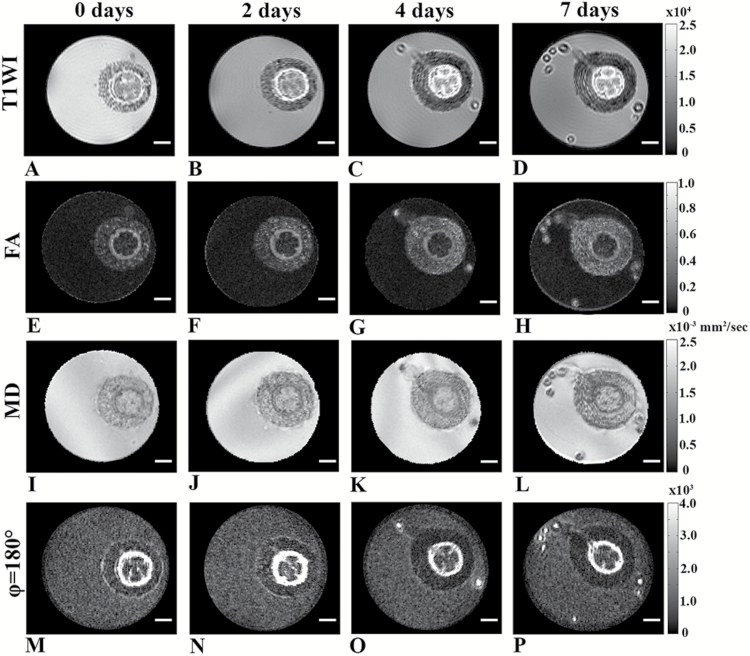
Time-course of adventitious root formation in 5-week-old plants by live MRI imaging (in-plane resolution of 61 µm^2^). (A–D) T1-weighted images, (E–H) FA images, and (I–L) MD maps obtained from DTI. (M–P) Zero mixing time d-PGSE MR images for *φ* of 180° map. All scale bars are 1 mm.
